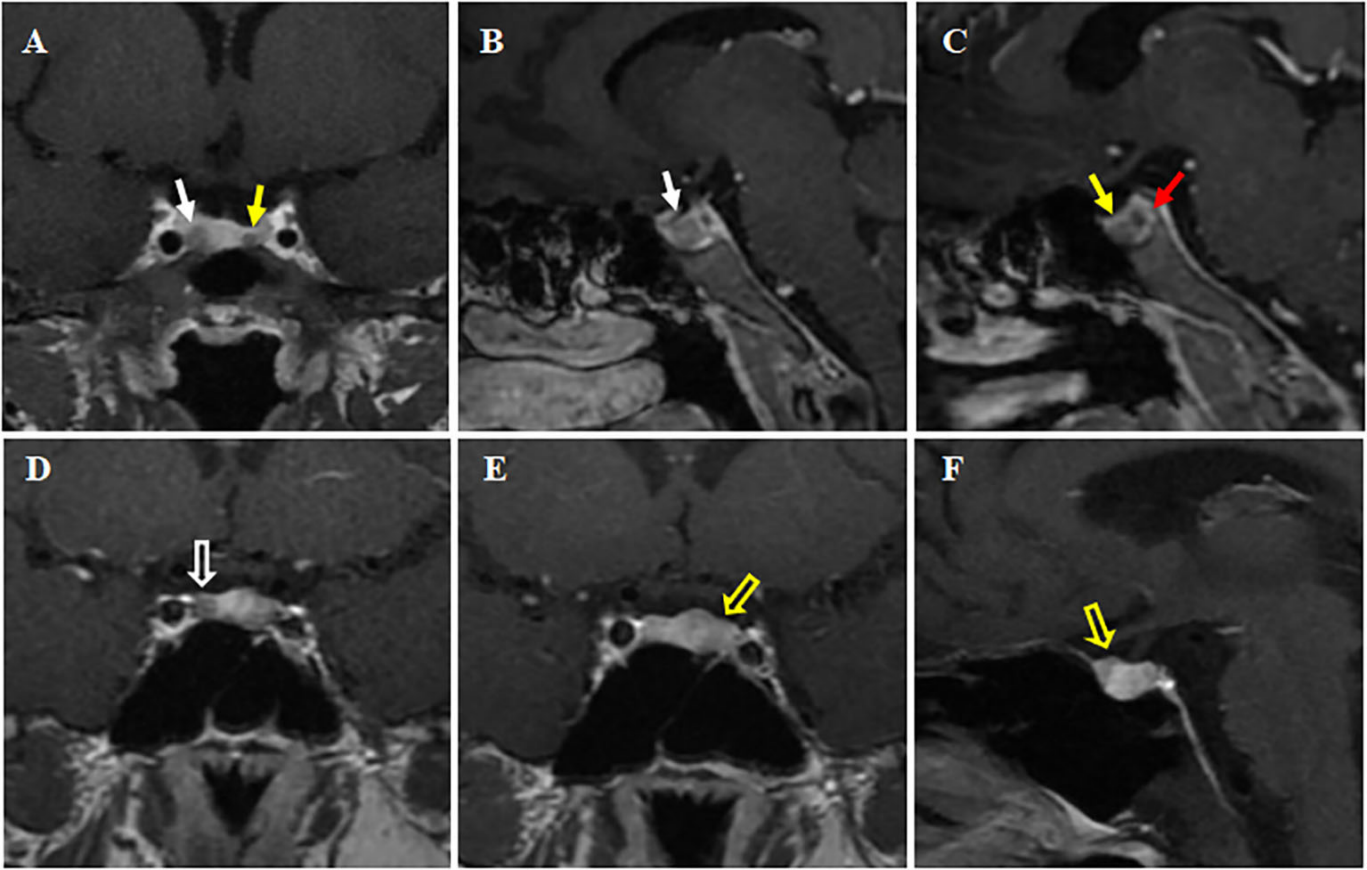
Preoperative dynamic contrast-enhanced MRI of the pituitary. (A-C) Case 1. The pituitary gland demonstrates heterogeneous enhancement, with bilateral areas of hypointense signal. Larger (about 11.3 × 5.5 mm) on right side (white solid arrows, (A, B); Smaller (about 3 × 3.5 mm) on left side (yellow solid arrow, A, C). A red solid arrow points to a non-enhancing area, representing a posterior pituitary cyst (C). (D-F) Case 2. A rounded hypo-enhanced signal about 5 mm in diameter is seen on the right side (white hollow arrow, in D), and a patchy area of reduced enhancement is observed on the left anterior side (yellow hollow arrows, in E, F). All A-F are post-contrast T1-weighted sequences. Coronal views in (A, D, E); Sagittal views in (B, C, F).
